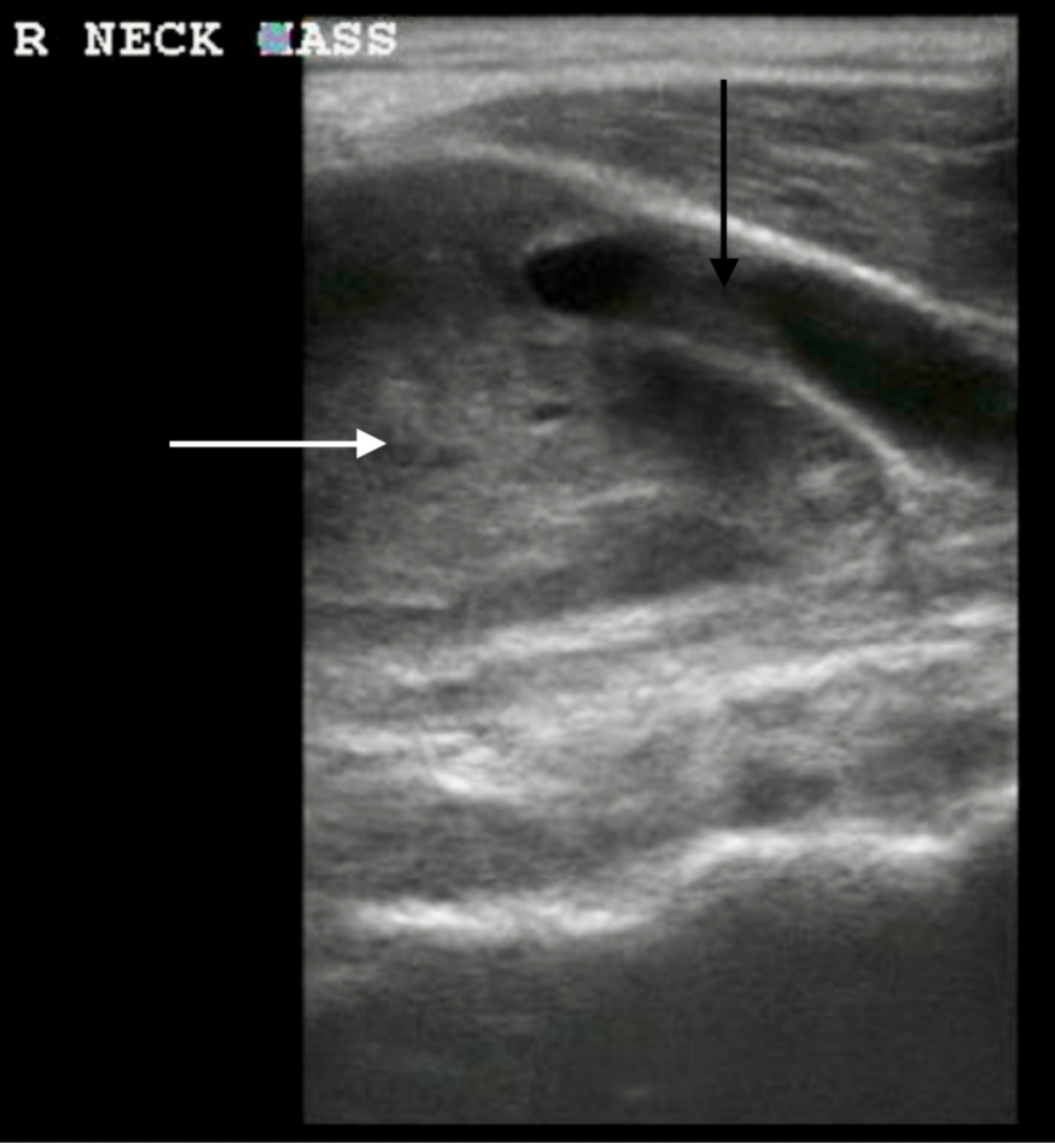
A bedside ultrasound demonstrating a homogenous neck mass (white arrow) at the level of the right carotid artery bifurcation (black arrow).
